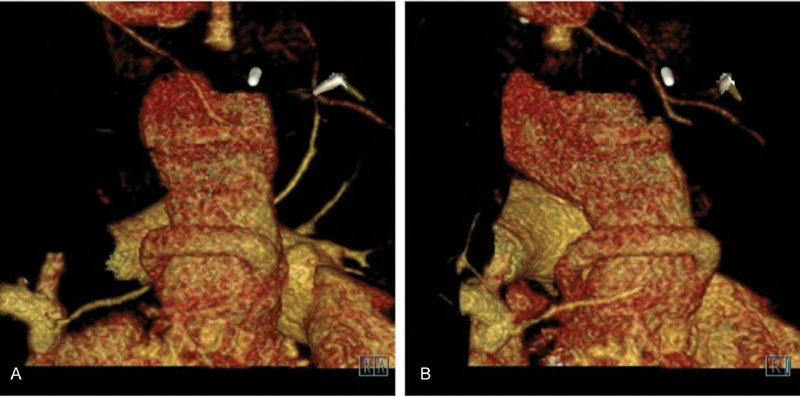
Computed tomography—three-dimensional reconstruction images: The 10 mm prosthetic graft is seen routed to the right side of the graft and anastomosed to the anterior surface of the tube graft (
